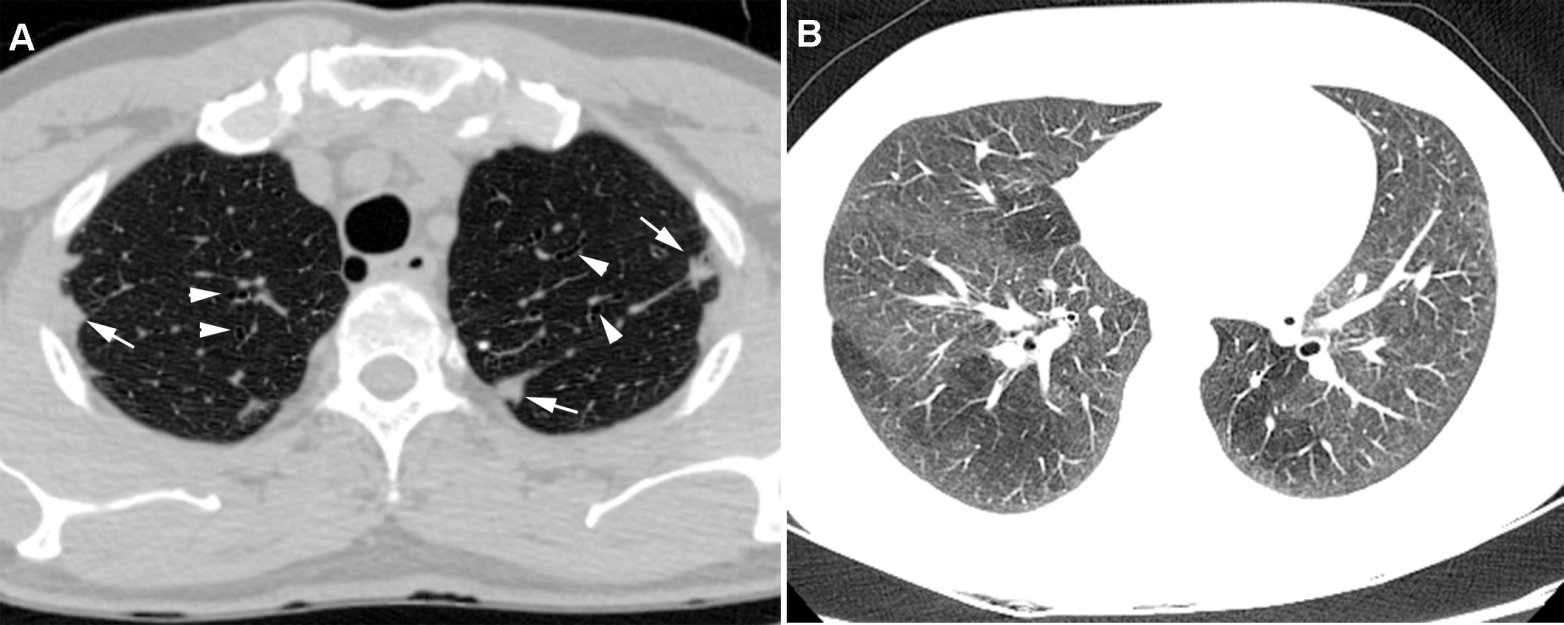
Transverse CT scans of a 59-year-old man and a 25-year-old woman with HAM/TSP. a) Pleural thickening (white arrows) and bronchiectasis (arrowheads), bilaterally b) bilateral mosaic attenuation.
